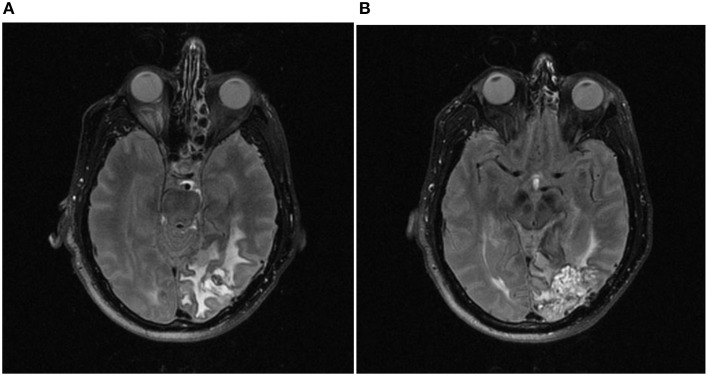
Two axial T2 FLAIR MR images showing the location of DY's lesion (1 × 1 mm in-plane resolution, 3 mm slice thickness; slice locations relative to the nasion are as follows: (A) −35 mm, (B) −26 mm). The lesion is confined mainly to the posterior regions of the right hemisphere (predominantly occipital, but also including the precuneus), and extending ventrally into temporal lobe regions.
